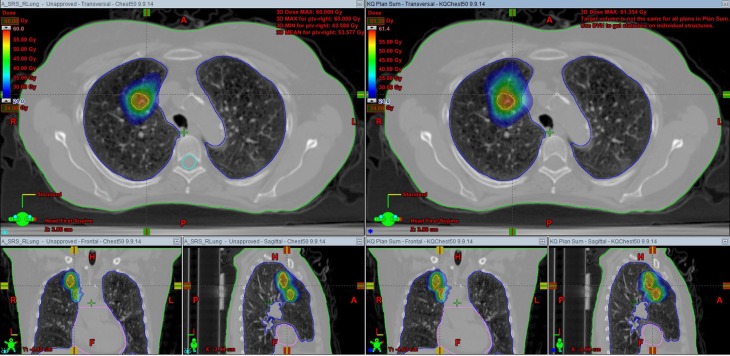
CT scans of the target, with a colorwash display of the dose (24 Gy and above) for a 48 Gy plan, showing the smaller spread of the intermediate dose distribution in the single-isocenter plan. Left, single-isocenter plan; right, multi-isocenter plan.
